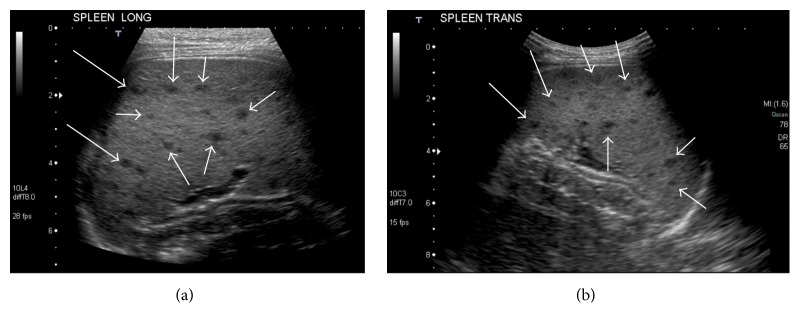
Longitudinal and transverse ultrasound images of the left upper quadrant demonstrate the spleen to be enlarged. Multiple small hypoechoic lesions (arrows) are seen within the spleen.
